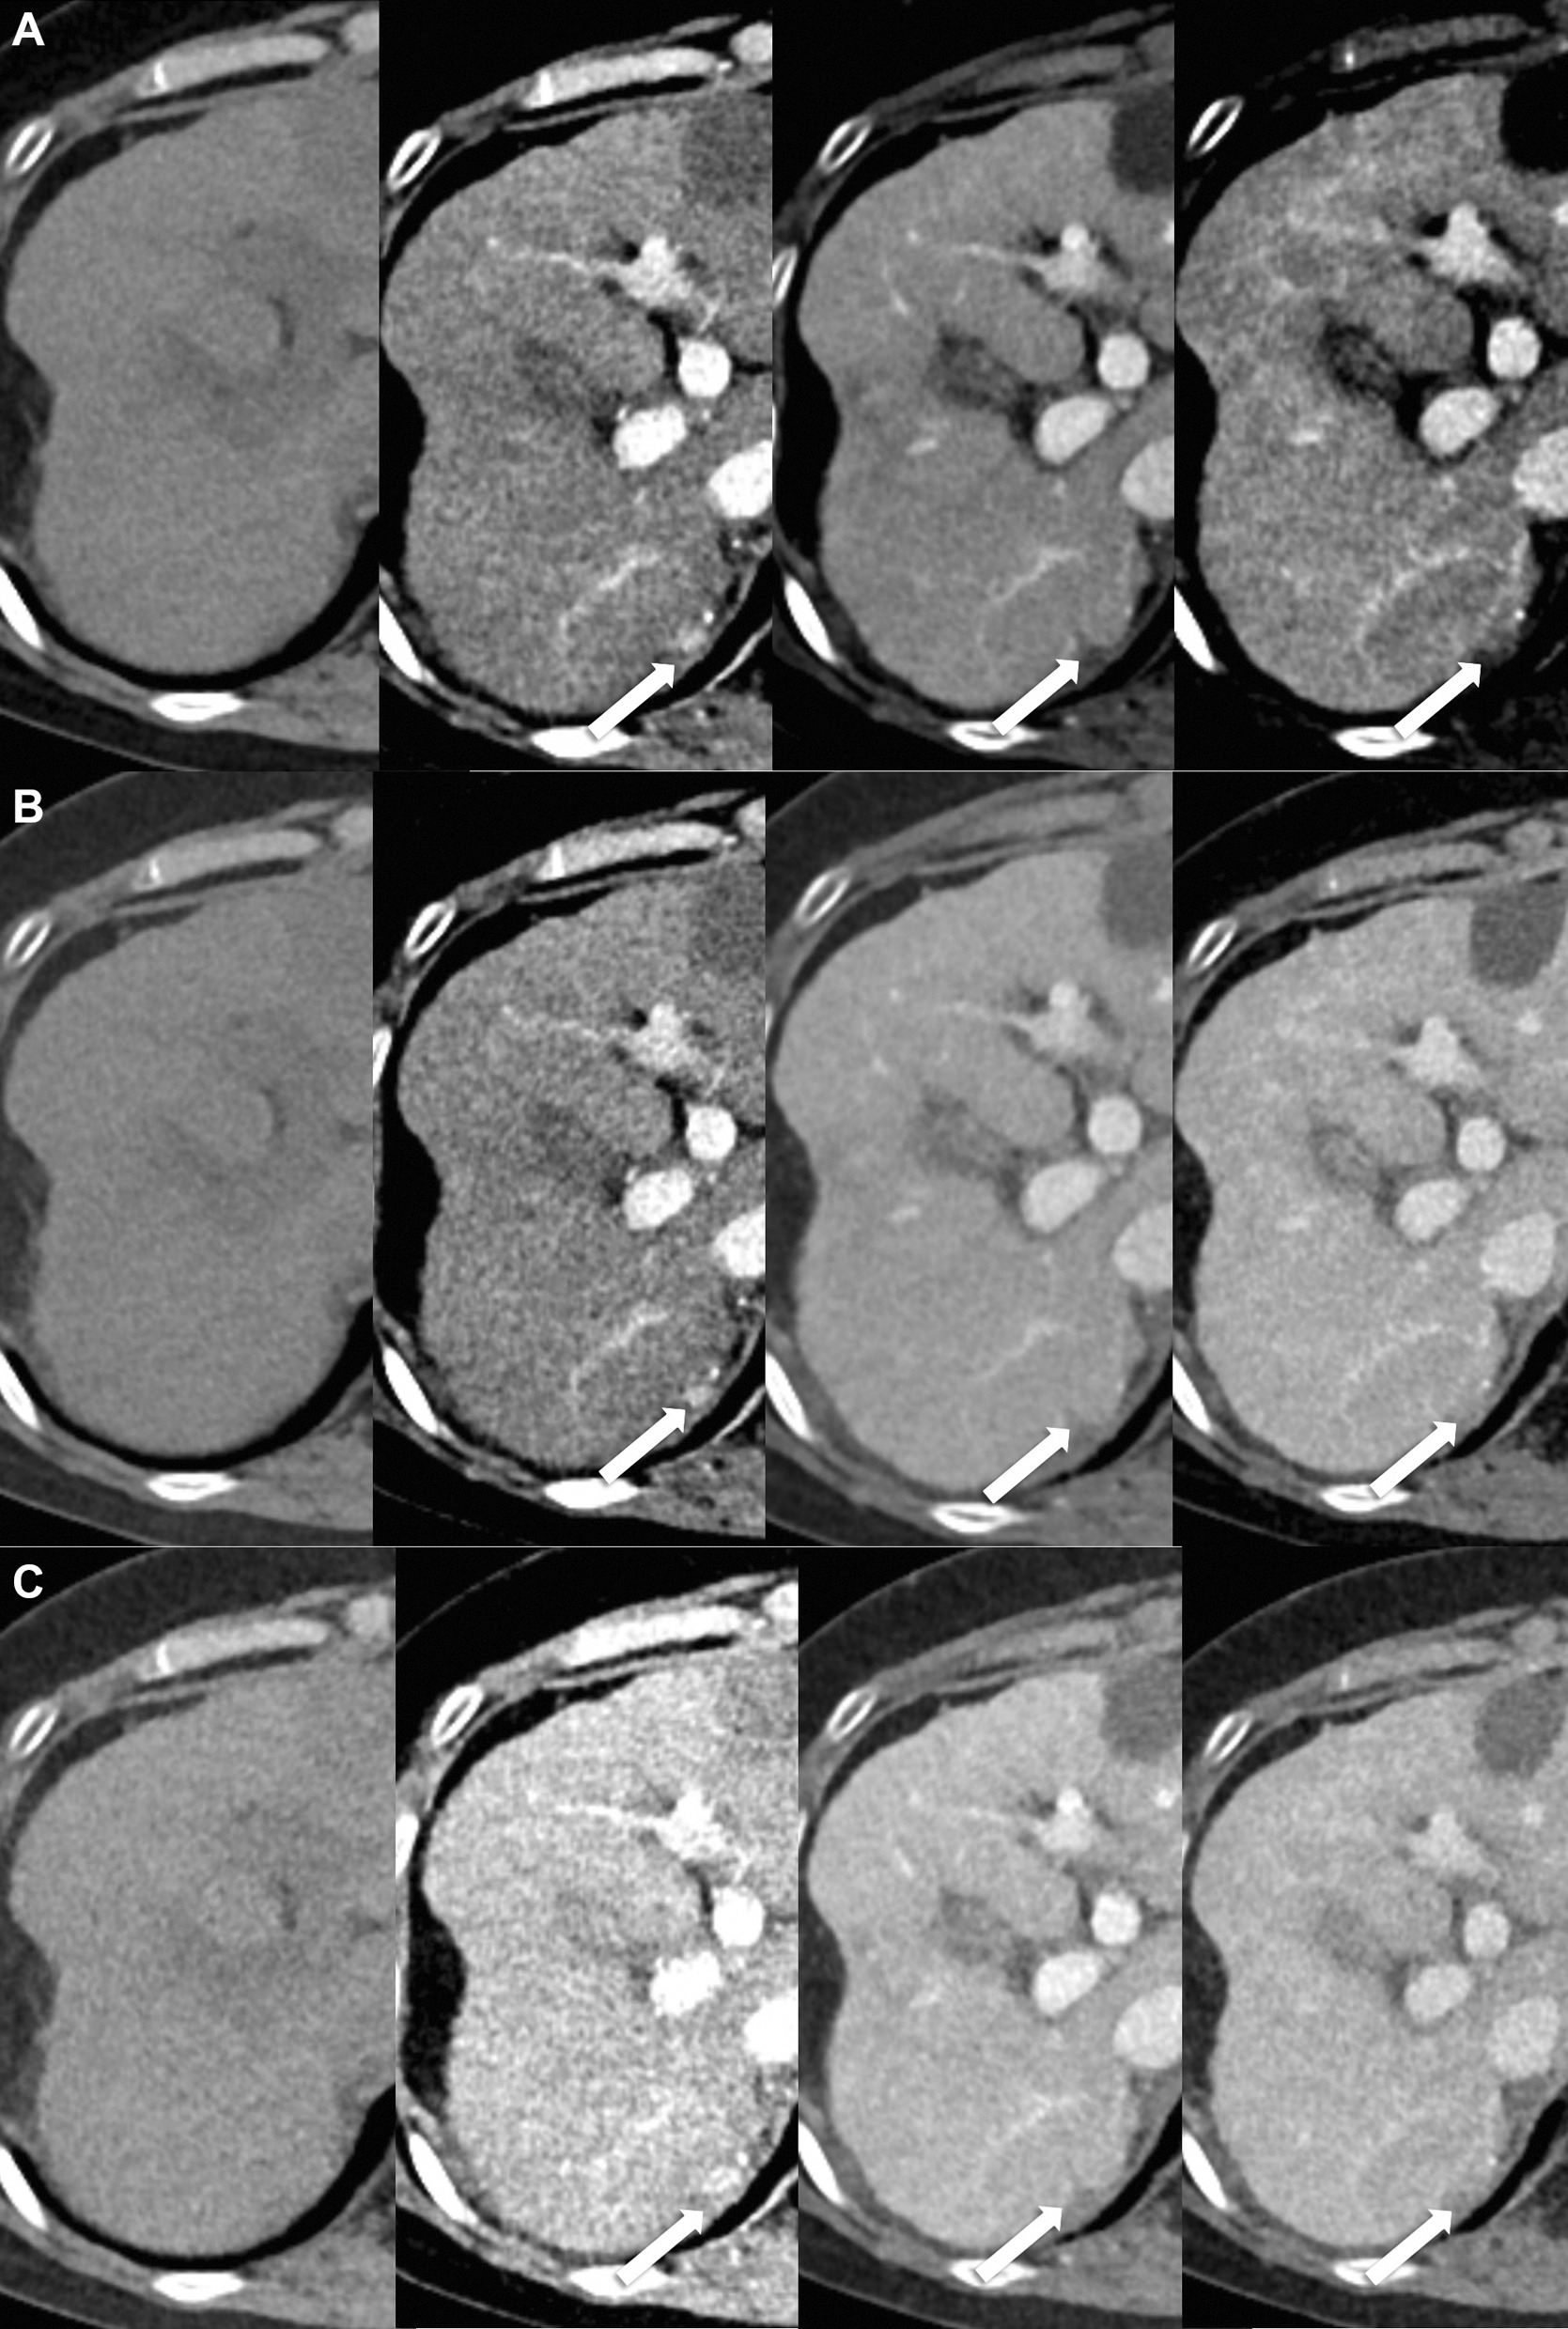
A 0.9-cm sized hepatocellular carcinoma in a 65-year-old woman with 20 kg/m^2^ body mass index. A In standard-dose CT (8.3 mSv), both reviewers gave a LI-RADS score of 4. B In low-dose CT (5.8 mSv), reviewer 1 gave a LI-RADS score of 4 but reviewer 2 could not detect the lesion. C Both reviewers could not detect the lesion in ultra-low-dose CT (2.5 mSv)
